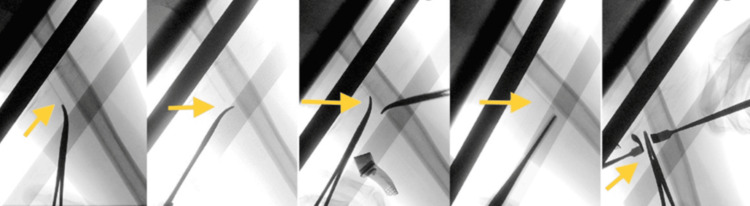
Implant removal procedure using fluoroscopy The image shows the orthopedic surgeon using a fluoroscope, a specialized X-ray machine, to enhance visualization during the removal process.
